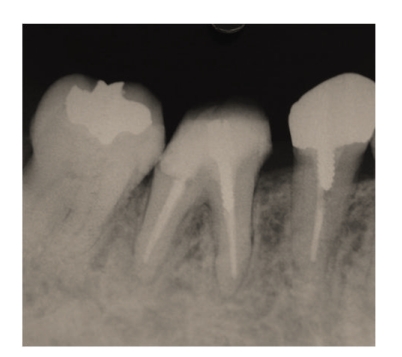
Radiography 10 weeks after the initial visit showing that the radiolucency at the apex had decreased.
